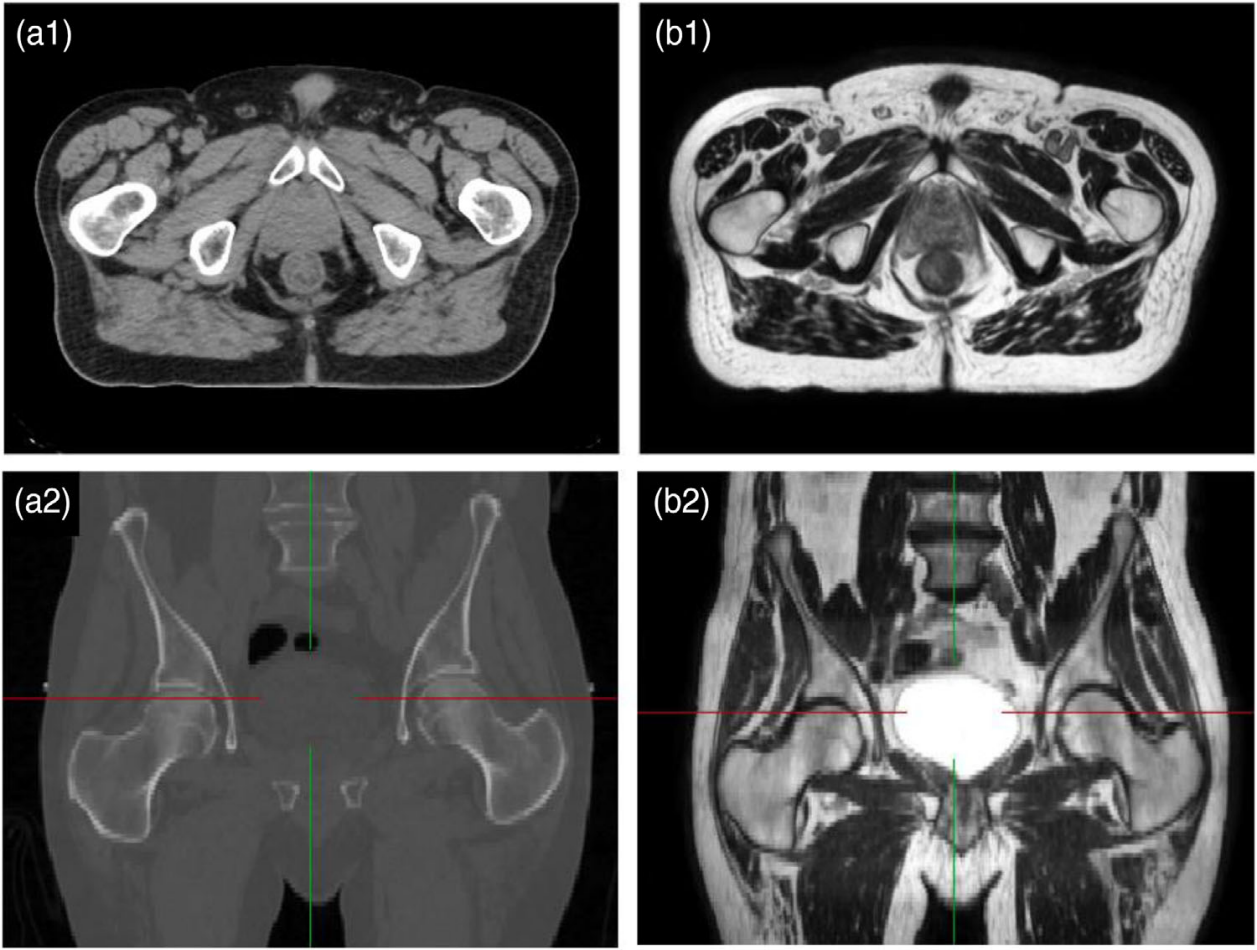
A corresponding pair of axial and sagital CT images shown in Panels A1 and A2 and the corresponding calculated synthetic MRI images shown in panels B1 and B2 for pelvic case.
